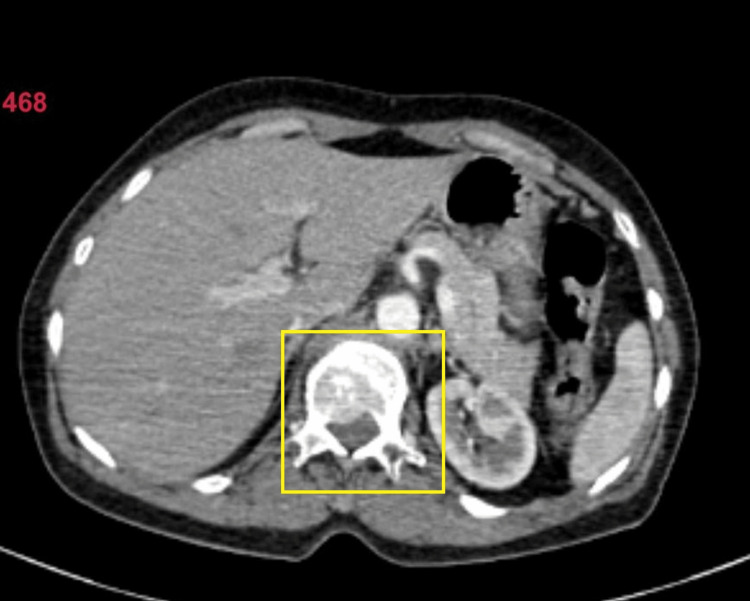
Computed tomography image showing lytic lesion of the vertebra (axial view)
